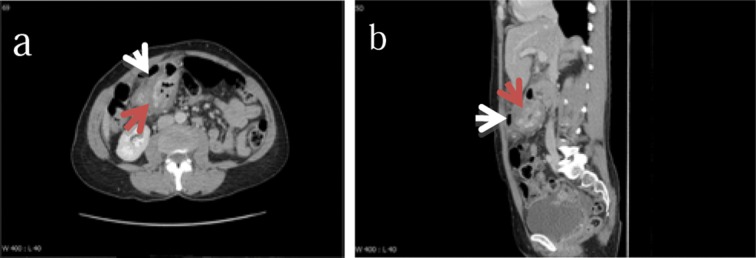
Axial and sagittal contrast enhanced computed tomography of a thickened and heterogeneously enhancing mass in the gastric antrum (dark arrow) with surrounding soft tissue stranding and localised free air anterior to the mass (light arrow)
